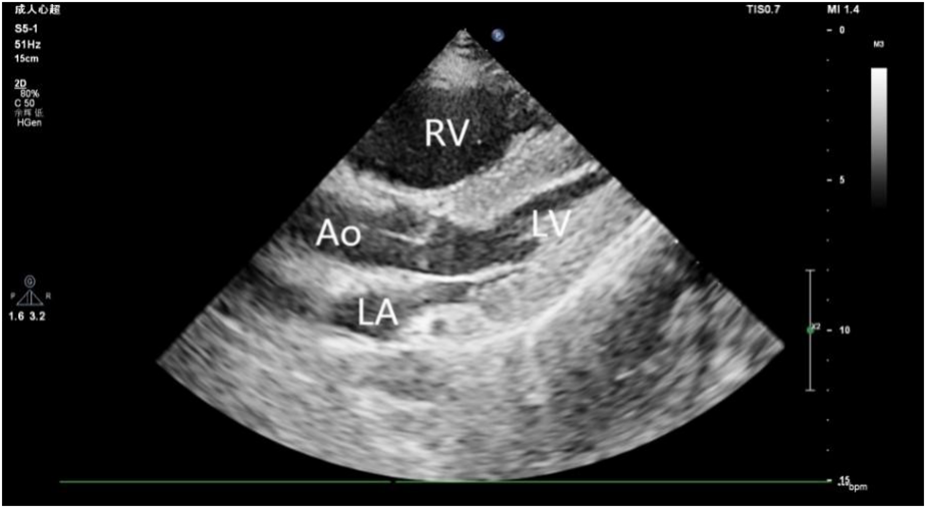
Ultrasound image reveals an enlarged right ventricle and underfilled left ventricle. RV, right ventricle; LV, left ventricle; LA, Left atrium; AO, Aorta.
